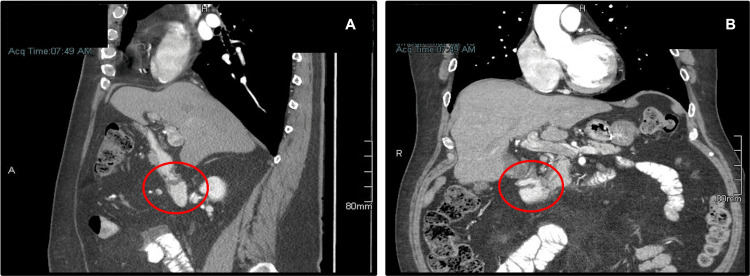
CT scan of the abdomen showing stricture at the level of the proximal second part of the duodenum described as a “duodenal band” A: sagittal view; B: coronal view
